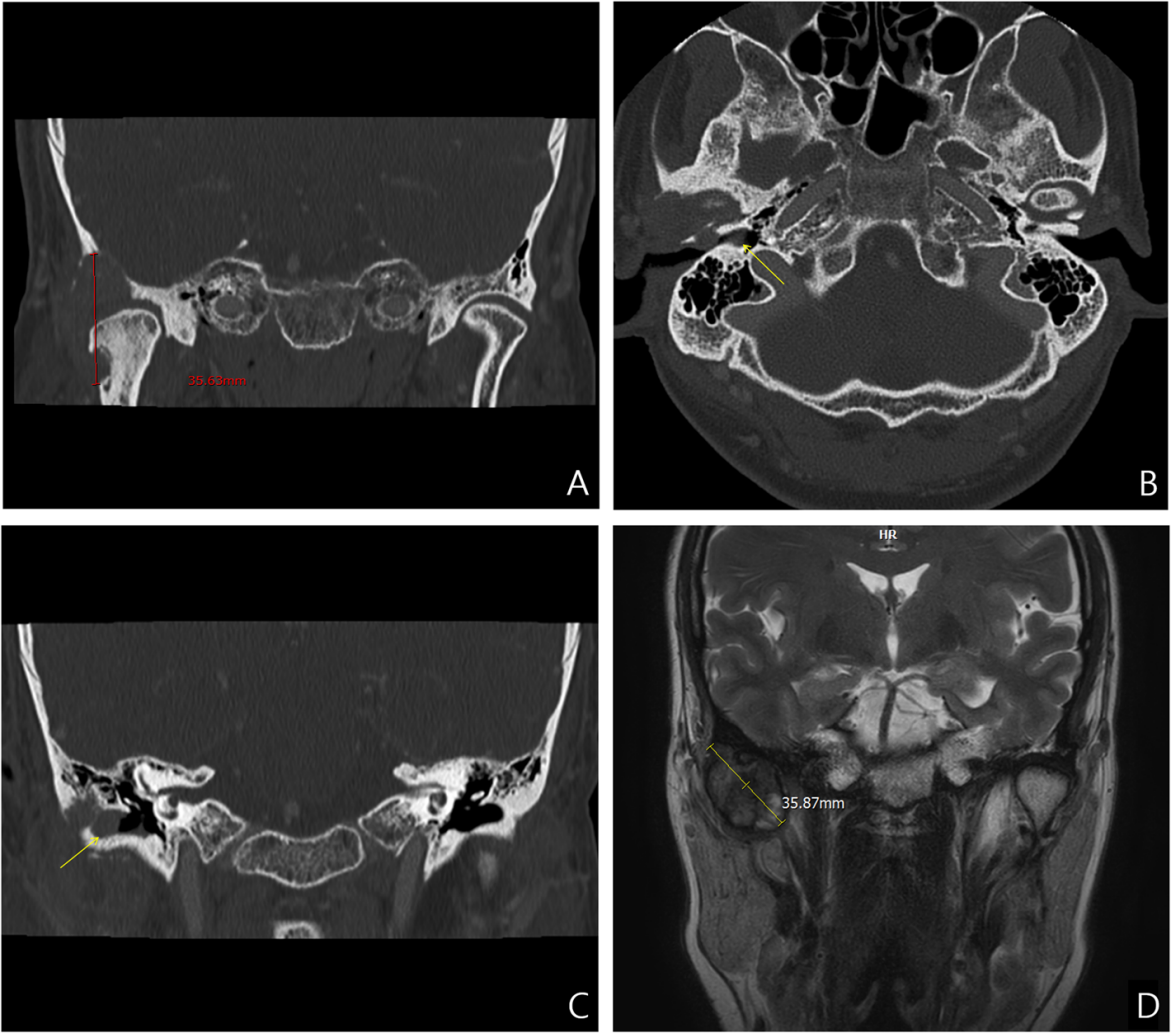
Preoperative computed tomography A coronal view shows erosion of the skull base temporal bone and the moth-eaten appearance of the right mandible neck. The mild thickening of the tympanic membrane (B, arrow) and fluid collection in the external auditory canal (C, arrow) were tentatively diagnosed as external otitis due to an error in the interpretation of the images. Preoperative magnetic resonance imaging D coronal view shows an inhomogeneous, multi-lobulated mass with an intermediate signal
